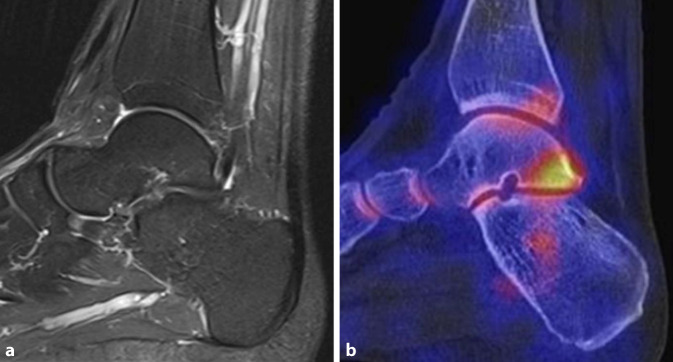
## SPECT/CT bei Koalitionen und Arthrodesen
